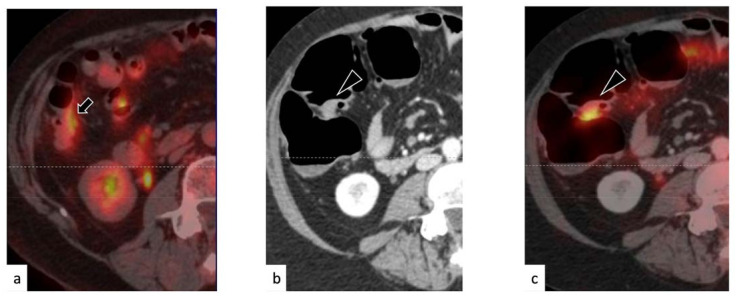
False-positive finding at positron emission tomography/contrast enhanced X-ray computed tomography PET/ceCT. The PET/ low dose (ld)CT (a) showed diffused, low-grade fluorodeoxyglucose (FDG) uptake within the ascending colon, which was deemed unspecific. However, the PET/ceCT (b,c) identified a seemingly nodular formation in the colic wall, with intensive FDG uptake. At further follow-up, the uptake disappeared without treatment, and the nodular formation was revealed as an artefact, due to abdominal distension.
